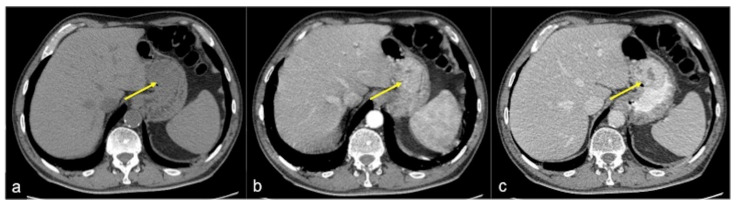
CTA multiphasic study of gastric GIST. In the pre-contrast (a), arterial (b) and venous (c) phases a large vascularised lesion in the gastric body with massive haemorrhagic lumen inundation is visible (a–c, arrow).
